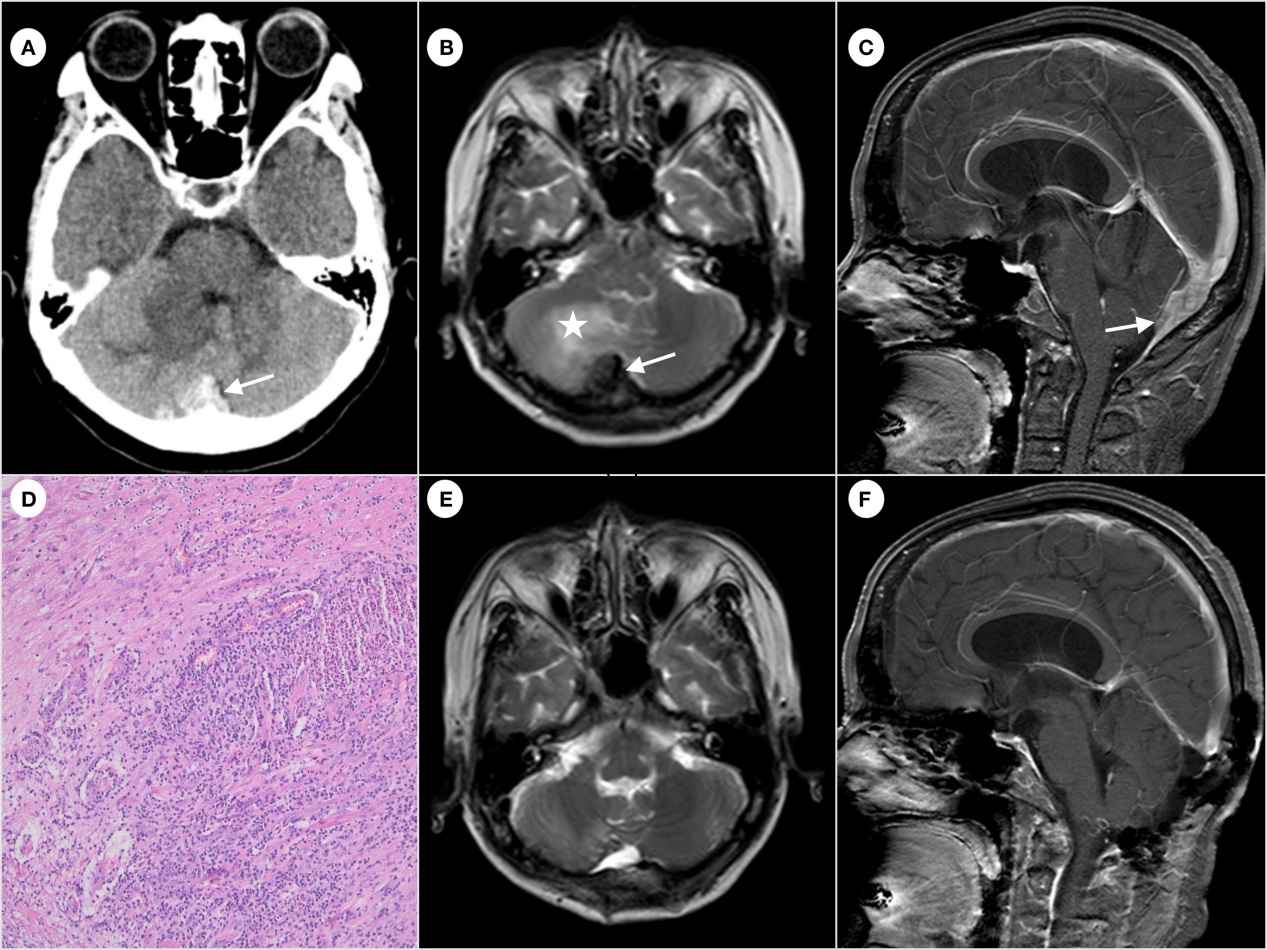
Computed tomography of the brain showing irregular high-density mass (arrow) below the occipital plate (A). Hypointense (arrow) on T2-weighted magnetic resonance imaging and edema (star) observed near the right cerebellar hemisphere (B). The enhanced scan shows significant enhancement and a dural tail sign (arrow) (C). Formation of granuloma and central stellate microabscess. The image was acquired at 100× magnification (D). The follow-up MRI shows the disappearance of the residual lesion in the surgical area (E, F).
